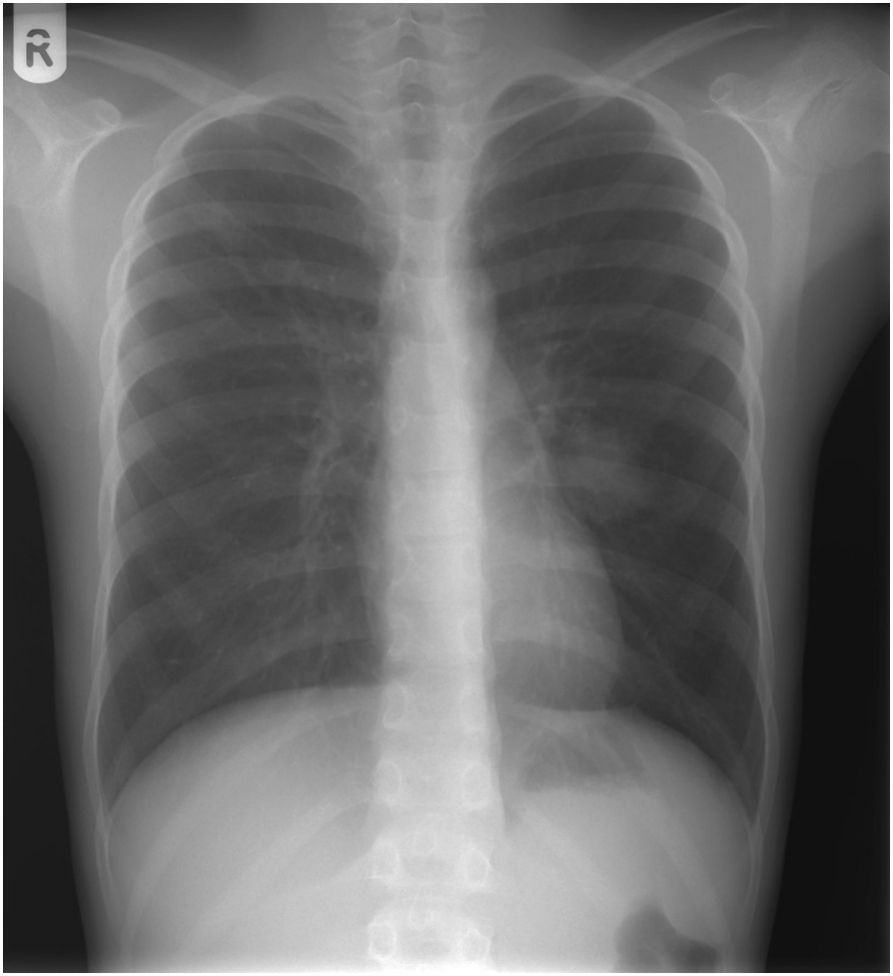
Chest X-ray showed a homogeneous soft tissue mass 20×30mm in the lingual area.
